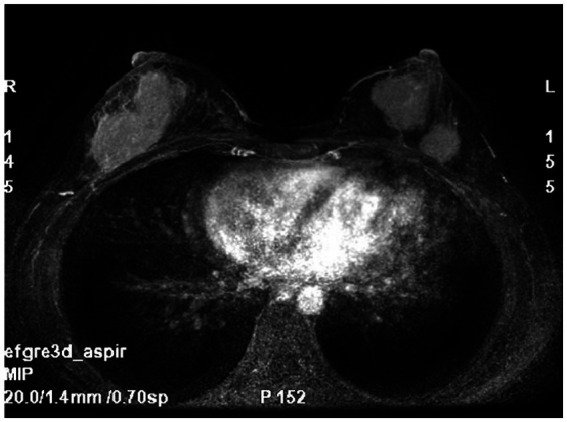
Breast MRI: bilateral breast mass, irregular shape, irregular edge, marked enhancement.
